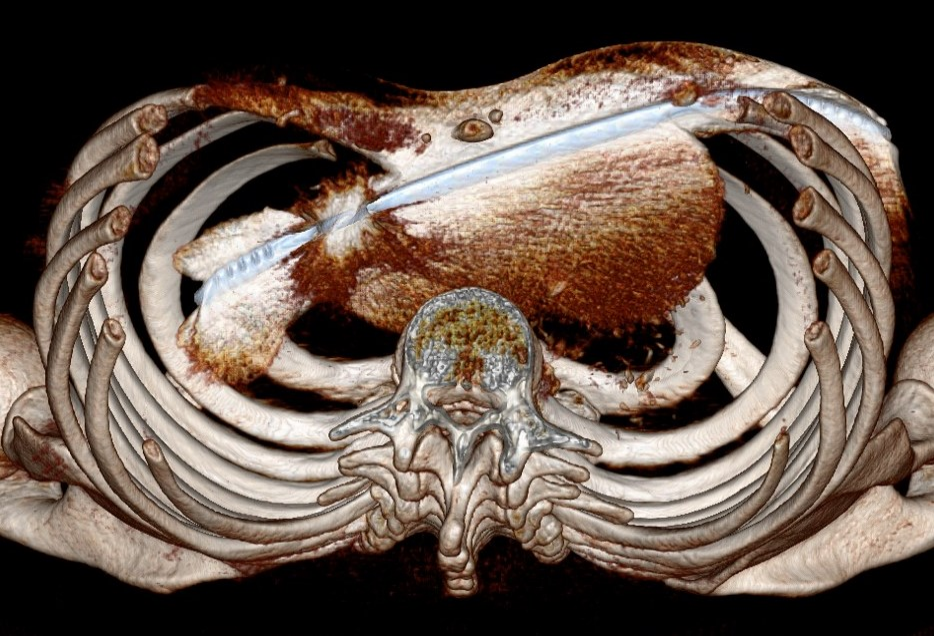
Three dimensional computed tomography reconstruction of intrathoracic pectus bar displacement on the side with the stabilizer attached, compressing the heart and lung.
